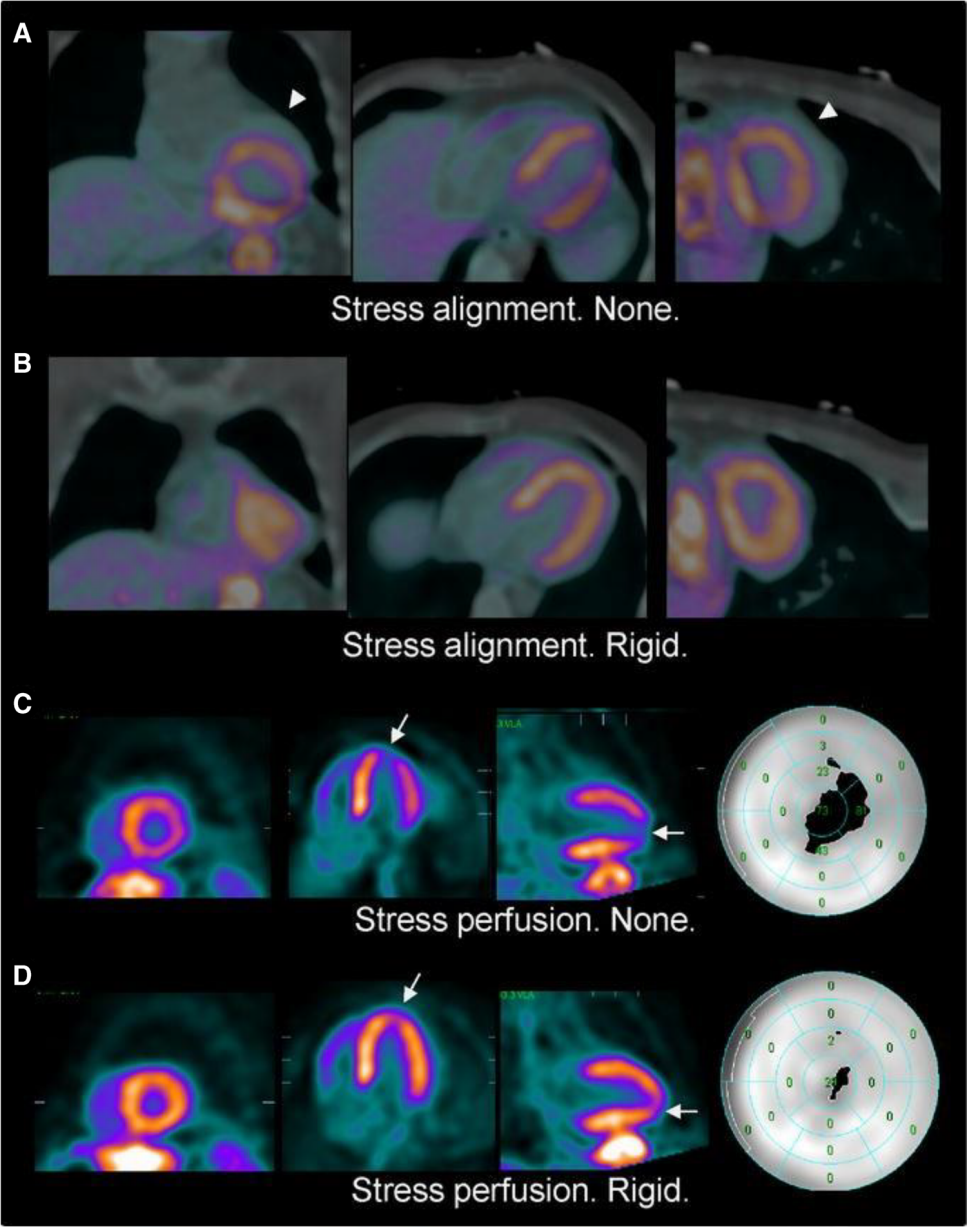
False-positive nuclear scan due to the CT registration error resolved by the automatic correction in a 44-year-old male presenting with shortness of breath. PET and CTAC images fused before alignment (A) and after rigid alignment (B). Misalignment is indicated by the white arrow head (A). Stress perfusion PET images before alignment (C) and after alignment (D) and polar maps are shown with the white arrow indicating the perfusion artifact due to misalignment. Quantitative total perfusion defect (TPD) was 11% (C right), when no correction for alignment was performed (ITPD after manual verification was also 11%) compared to a normal scan (TPD 1.8%) after automatic registration (D right). Invasive coronary angiography did not show significant coronary artery disease (Reprinted with Permission of Springer from [71], no changes were made)
